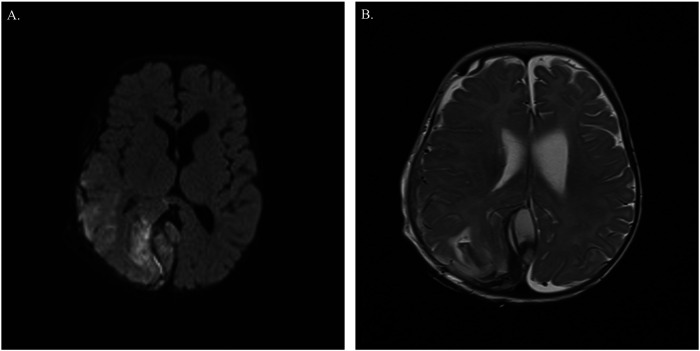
(A) CT brain: an axial unenhanced CT image of the brain shows a large multicompartmental intracranial hemorrhage (ICH), including subdural, subarachnoid, and possible epidural hemorrhage, along with right parietal–occipital infarcts, midline shift, and subfalcine herniation. (B) MRI brain: an axial T2-weighted MR brain image demonstrates heterogeneous extra-axial hemorrhage within the posterior interhemispheric fissure and over the right parieto-occipital and right frontotemporal lobes. A small amount of heterogeneous subarachnoid/intraparenchymal hemorrhage is also seen in the right parieto-occipital lobes. There is moderate edema in the right parieto-occipital lobes, with moderate mass effect on the right lateral ventricle, resulting in leftward midline shift.
